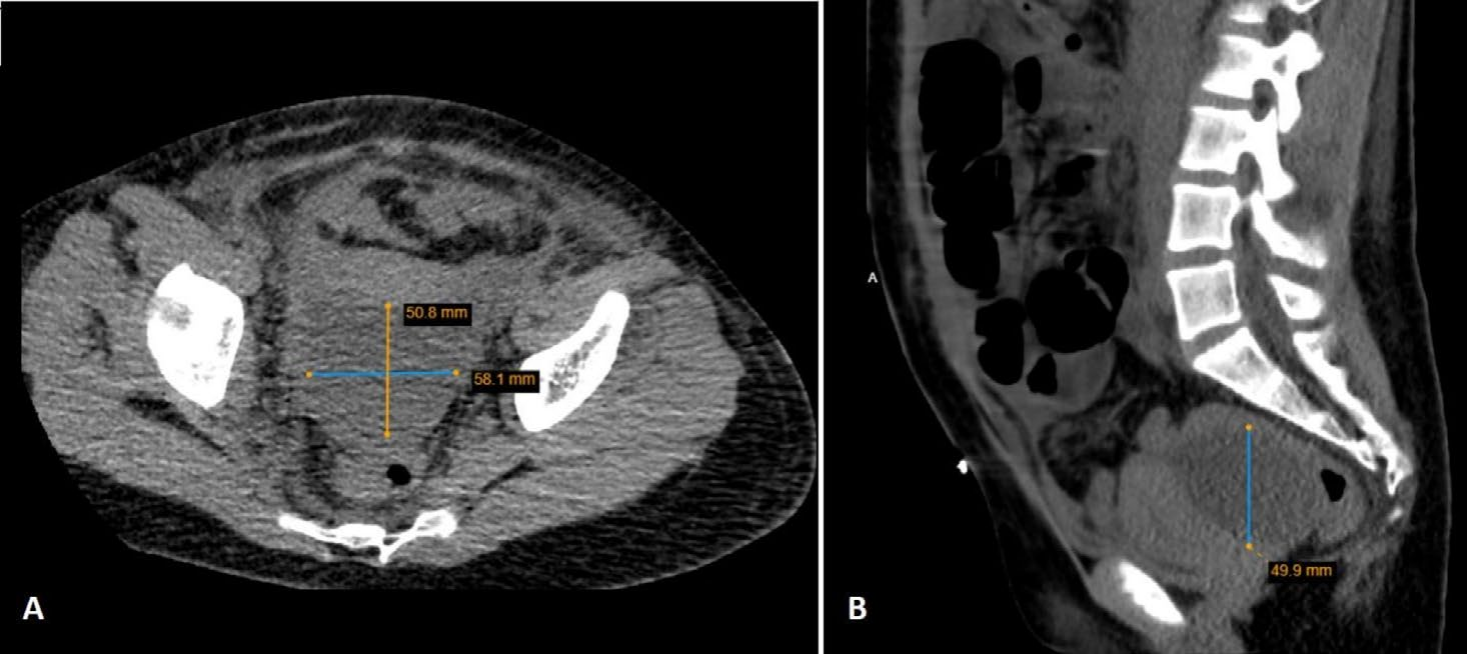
CT imaging of abscess formation in rectouterine excavation in transverse and sagittal plane (5 × 6 × 5.5 cm).
